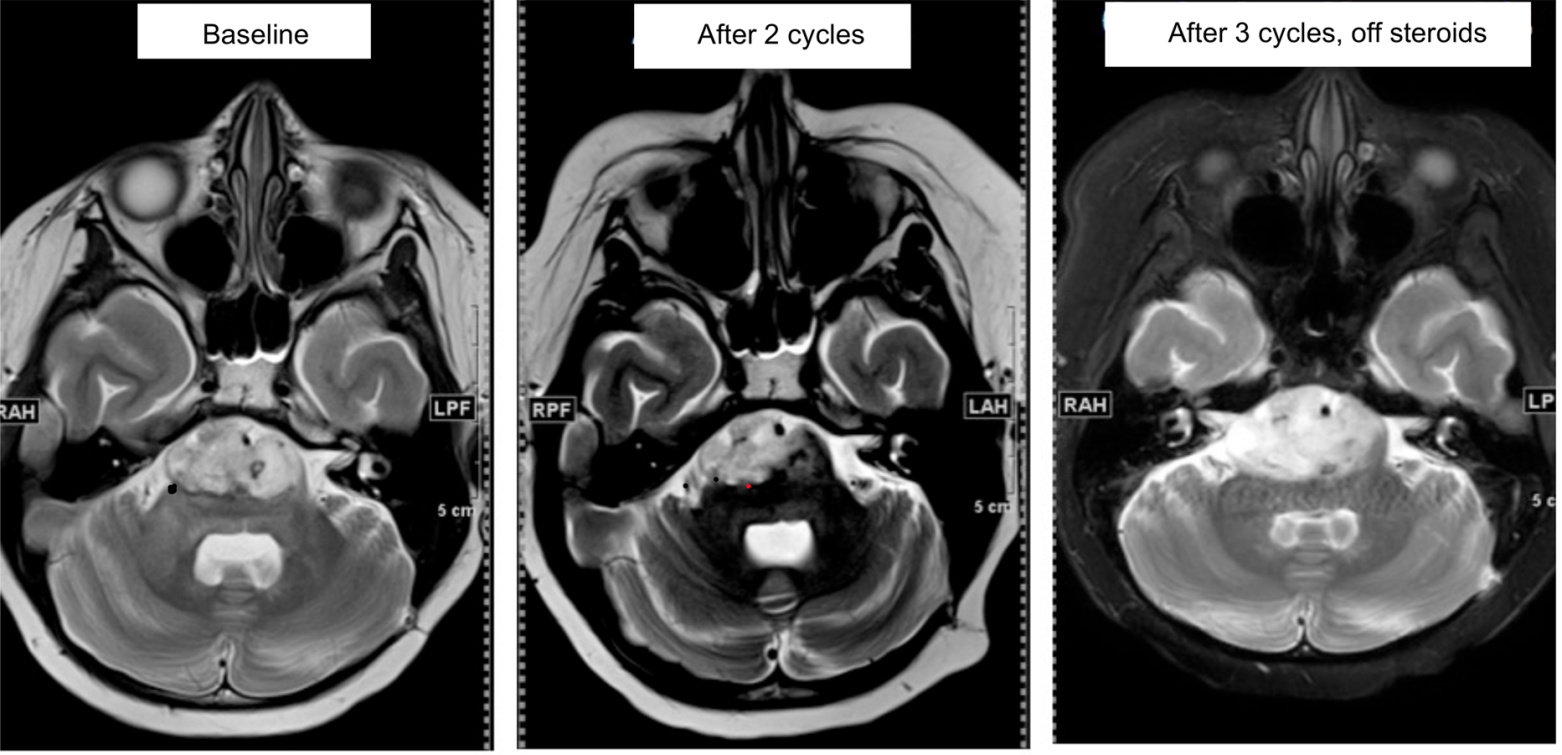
Radiographic tumor response to WP1066 treatment. Serial brain MRIs from a pediatric patient with progressive DIPG (AflacST1901-01) who had completed a 2-week course of palliative focal re-irradiation to the tumor 12 weeks before starting study treatment show 33.8% reduction in tumor volume after 2 cycles of WP1066 treatment compared with baseline MRI obtained 2 days before the start of study treatment (15.48 cm^3^ to 10.25 cm^3^). The patient was successfully tapered off high-dose corticosteroids and improved clinically, from being wheelchair bound to walking with assistance. Subsequent brain MRI performed after 3 cycles of WP1066 while off steroids for 4 weeks (pre–cycle 4) showed a 61.8% increase in tumor volume from baseline (25.05 cm^3^), but no increase in intratumoral blood perfusion, suggesting that the increase in tumor volume was due to treatment-related changes. Because of the increase in tumor volume, the patient was taken off protocol therapy per the study guidelines; however, the patient continued to exhibit clinical improvement followed by stability for an additional 6 months.
